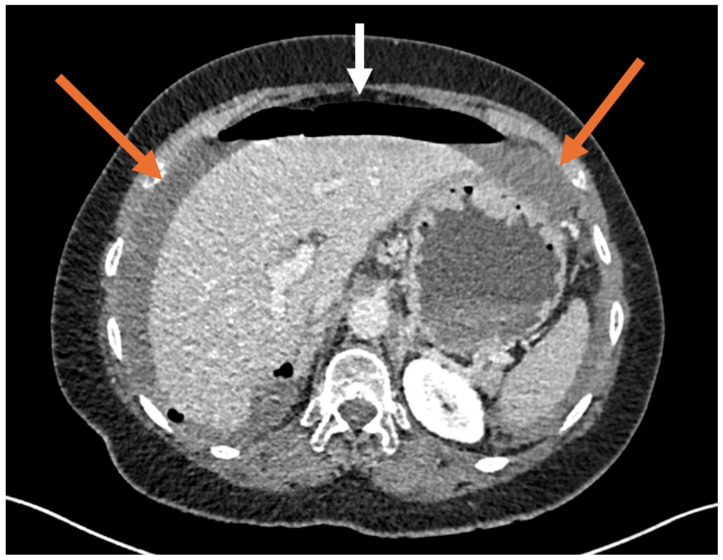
Given the persistence of epigastric tenderness and the absence of a cardiac etiology, an abdominal computed tomography scan was performed. It revealed diffuse pneumoperitoneum (white arrow), more prominent in the upper abdomen, along with diffuse thickening of the anterior gastric wall, thickened and infiltrated small bowel loops in the left flank and iliac fossa, and a moderate amount of intra-abdominal fluid (orange arrows), findings suggestive of a gastric perforation. The patient underwent emergency laparoscopic surgery, which confirmed a gastric perforation. A primary gastric suture repair (raffia) and peritoneal lavage were performed. Postoperative recovery was uneventful.
